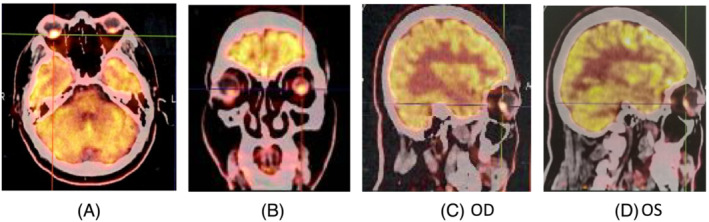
Positron Emission Tomography/CT scan on January 28, 2021. It showed the hotspots increasing fluorodeoxyglucose (FDG) uptake in the regions of the right (SUVmax = 4.8) and left (SUVmax = 5.6) eye funds, suggestive of a metastasis of malignant in both choroids symmetrically from lung cancer.
